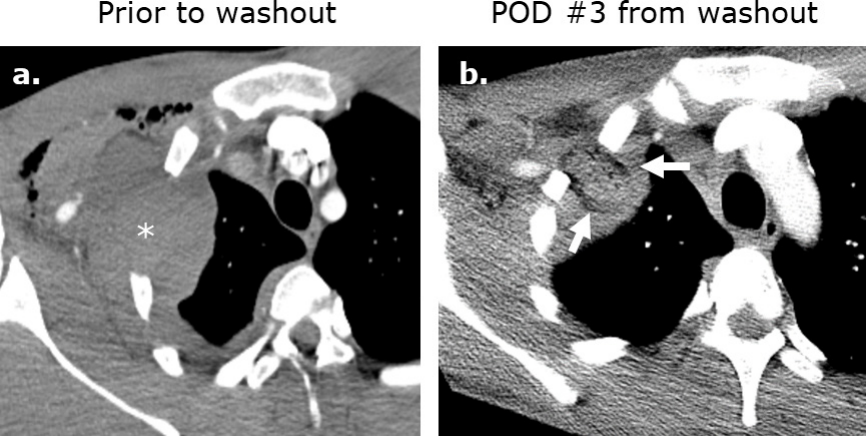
Surgicel mimicking abscess formation following washout of an iatrogenic hematoma. (a) Axial intravenous-enhanced CT image obtained at post-operative day 9 following ^®st^ rib resection for thoracic outlet syndrome in a 19-year-old male with pain and tachycardia revealing a hematoma at the surgical site. Axial (b) and coronal (c) intravenous contrast-enhanced images obtained following washout of the hematoma reveals Surgicel in the operative bed.
